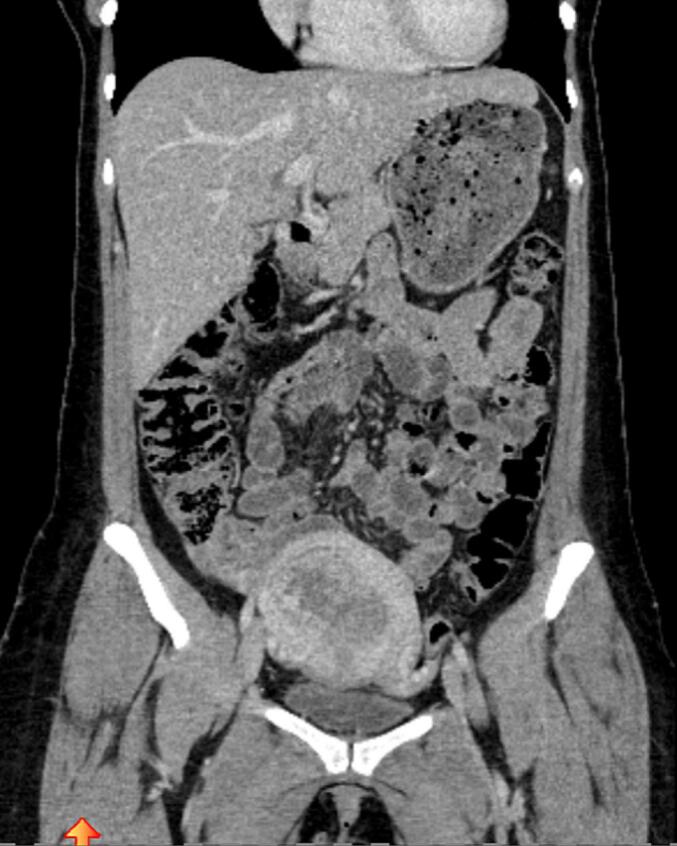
CT scan of the third patient right after severe vaginal bleeding started on the second day of EMA/CO chemotherapy.
